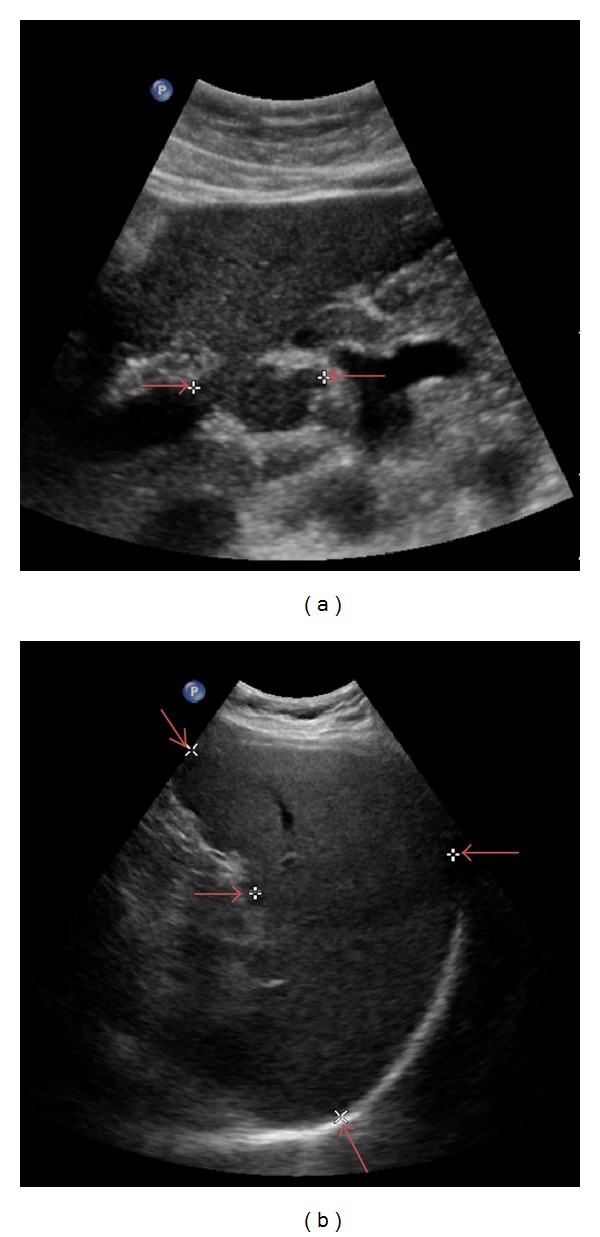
Abdominal ultrasound demonstrating lymphadenopathy and splenomegaly. (a) The lymph node diameter (3.1 cm) is demarcated by the arrows. (b) The spleen dimensions (18.3 cm × 9.3 cm) are demarcated by the arrows.
